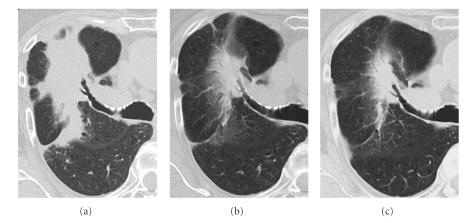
Serial CT images. (a) CT images at 15 months after SBRT show the mass-like consolidation enlarged at its dorsal side. (b), (c) CT images at 18 and 27 months after SBRT show that the mass-like consolidation gradually retracted.
